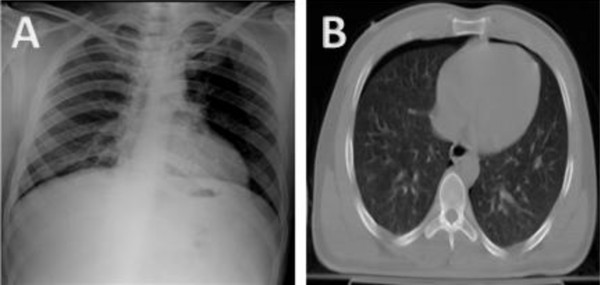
Anteroposterior chest X-ray and CT scan chest demonstrating "true occult pneumothorax". Anteroposterior chest X-ray revealing no evidence of pneumothorax (Panel A). CT chest performed immediately after X-ray revealing right sided pneumothorax (Panel B). Adapted from Omar et. al. [6].
